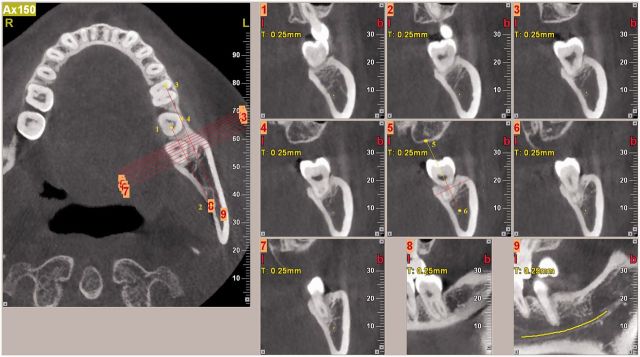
CBCT sections for quantitative analysis (experimental site before extraction). Typical lines on chosen sections to determine the position were shown. The reference line is between point 5 and 6. Lines for alveolar ridge width and height are marked on the reference line.
